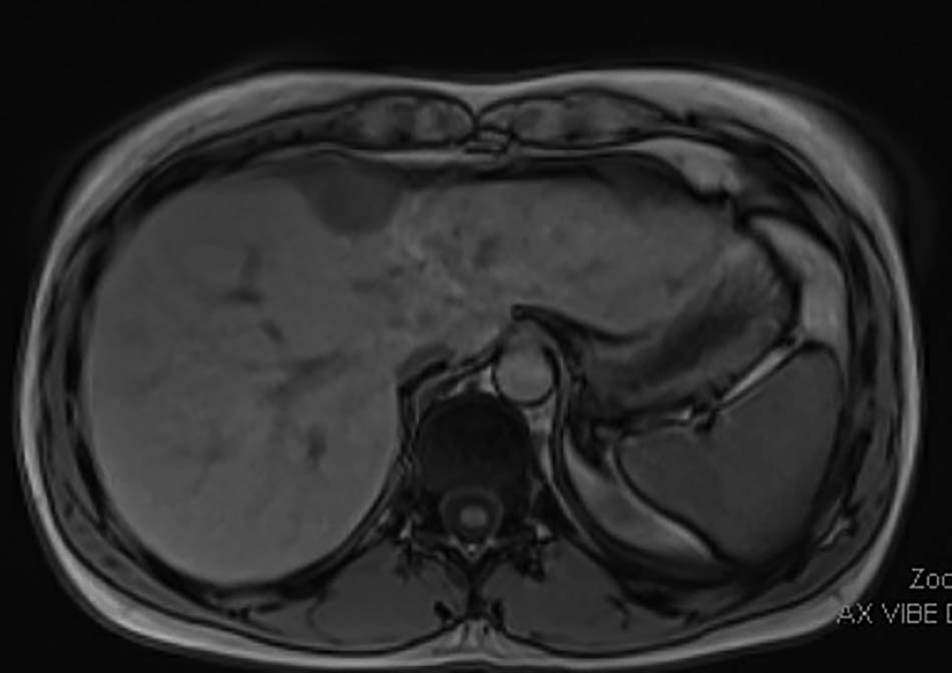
Pre-operative MRI image showing a 3.6 × 2.4 cm mass over segment IVa of the liver with possible parenchymal invasion.
